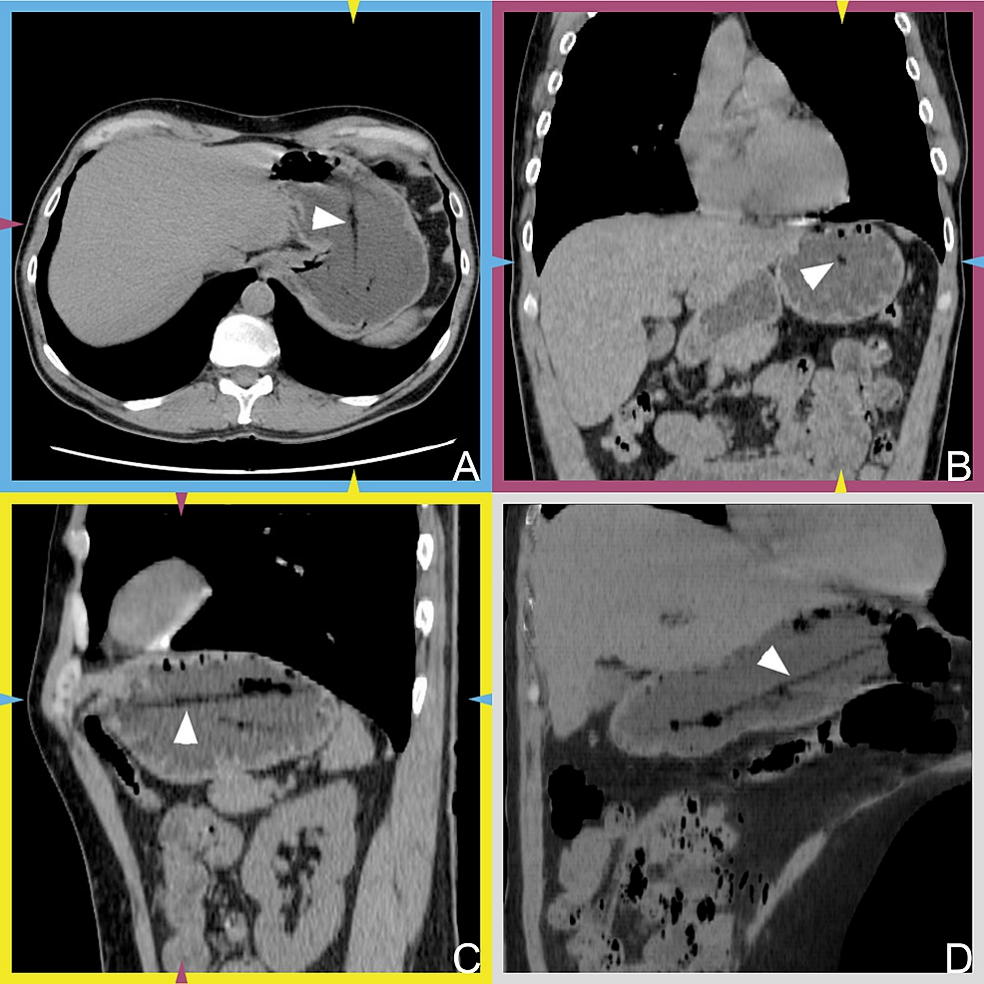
Multiplanar reconstructions of computed tomography scans. A–C: Multiplanar reconstructions of computed tomography images. A: axial plane, B: coronal plane, and C: sagittal plane. The elongated radiolucent area (white arrowheads) likely demonstrating the foreign body. The color-coded brackets and arrowheads indicate the corresponding planes. D: A minimum-intensity projection image generated from stacks, including sections of the foreign body. The oblique reconstruction was derived from sections acquired in a right recumbent position.
